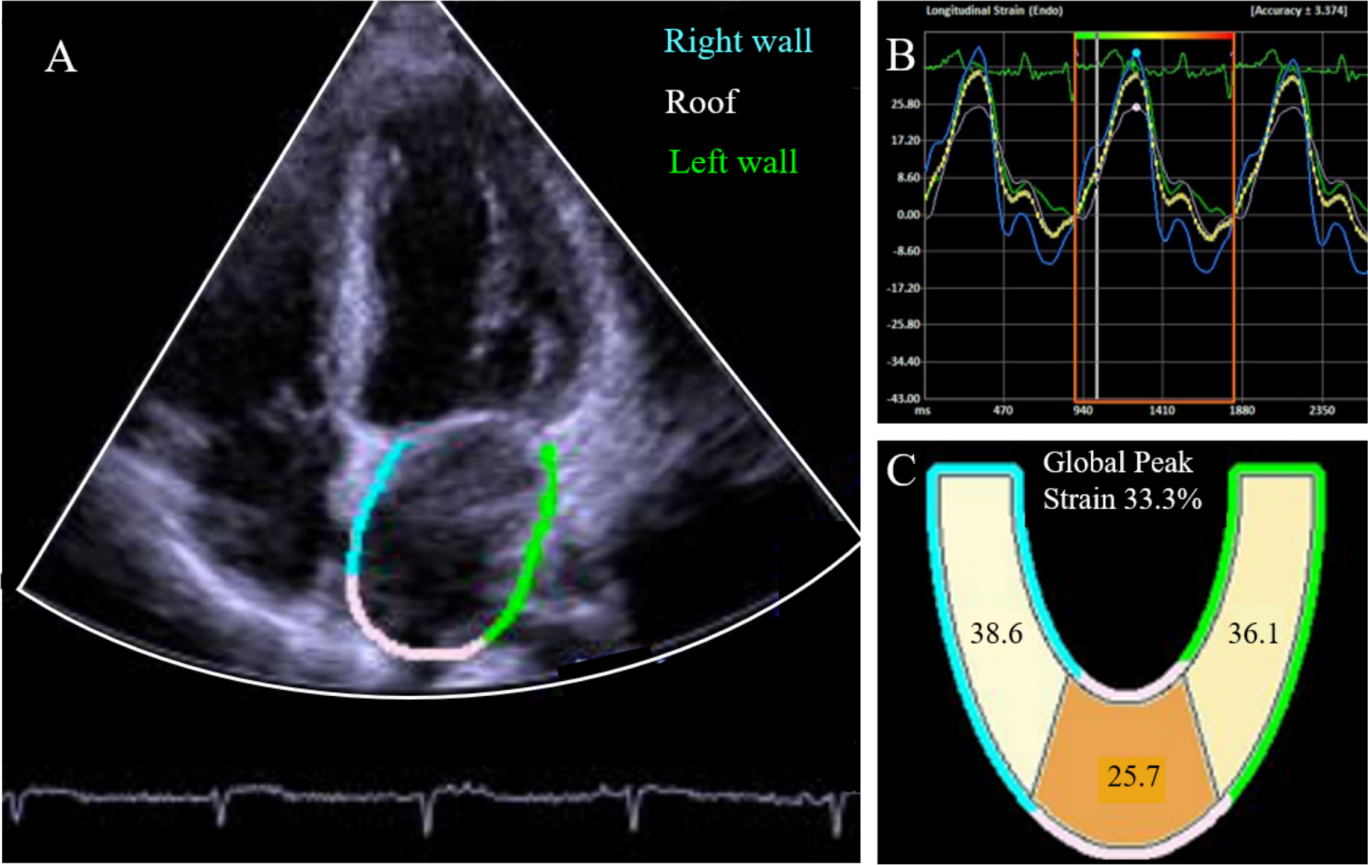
Exemplary measurement of left atrial global peak strain. Panel A: Apical 4-chamber view delineating the endocardial boundary of the left atrium in three sections (right wall, roof and left wall) as indicated by the respective colors. Panel B: Exemplary left atrial endocardial longitudinal strain curve derived from 2-D speckle-tracking. Panel C: Exemplary left atrial peak strain values for the three* different sections and derived left atrial global peak strain value*
